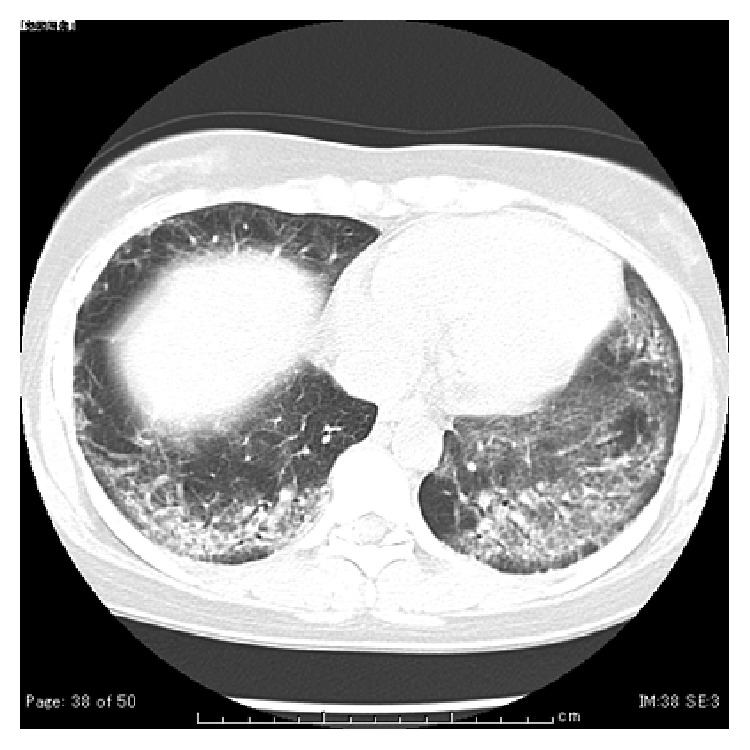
Chest-computed tomography on admission showing ground-glass opacity with partial honeycomb lung throughout the entire lung field.
